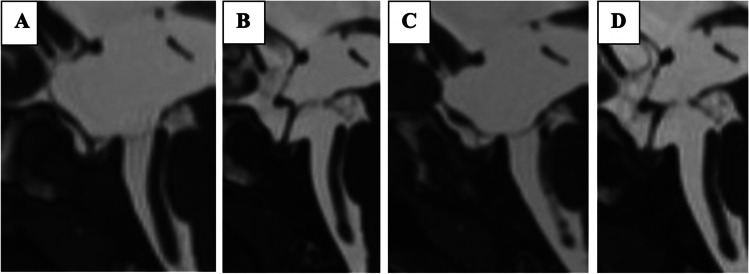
Pre-, 3-months and failure mid-sagittal CISS* magnetic resonance images of a 2 years old patient, who showed a preoperative Heidelberg score of + 2 (TVF + 1 and LT* + 1) (A), postoperatively 3 months later, the Heidelberg score went to -1 (TVF -1 and LT 0) (B), after 9 months, the patient showed hydrocephalic symptoms again and the Heidelberg score increased to + 2 (TVF + 1 and LT + 1) (C). 1 year after Re-ETV score dropped back to -1 (TVF -1 and LT 0) (D). *Constructive interference in steady state  Third ventricle floor *Lamina terminals
